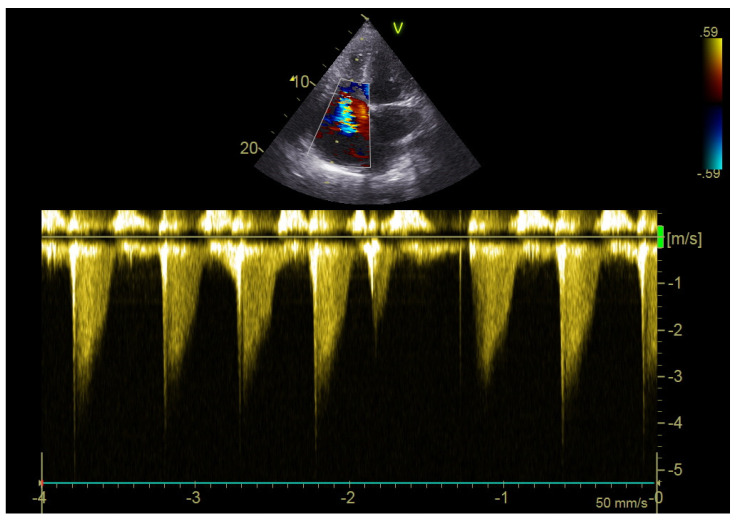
Continuous-Wave (CW) Doppler of tricuspid regurgitation from the 4-chamber view.
